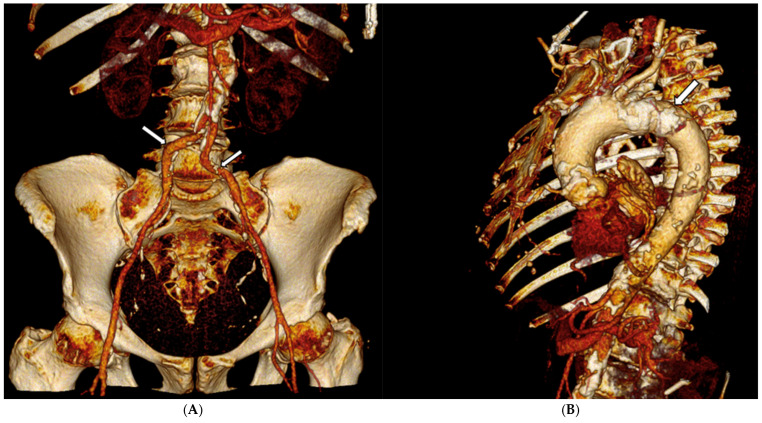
(A) Volumetric 3D CT angiographic reconstruction of the iliofemoral axis (arrows) demonstrating extensive calcified atherosclerotic disease; (B) Sagittal volumetric 3D CT angiographic reconstruction of the thoracoabdominal aorta reveals extensive calcified atherosclerotic disease indicative of porcelain aorta (arrow).
